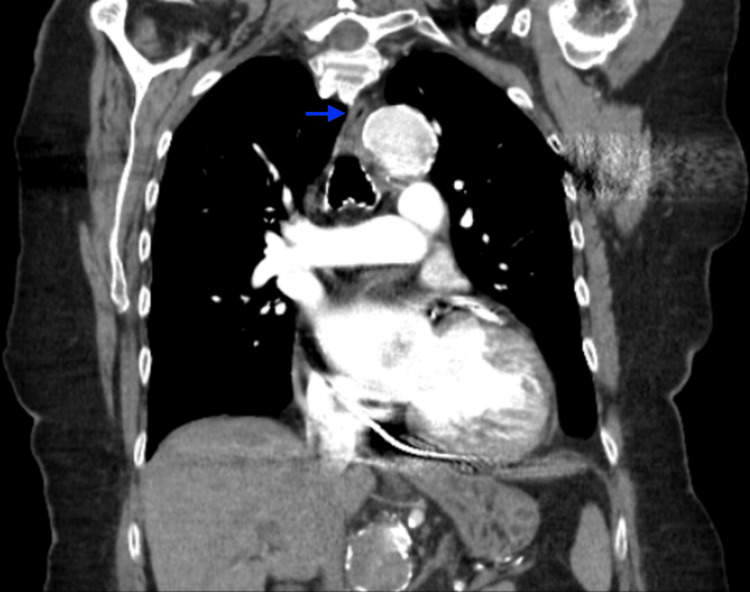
CTA in coronal view The blue arrow points to a compressed esophagus by an aortic aneurysm. CTA: computed tomography angiography
